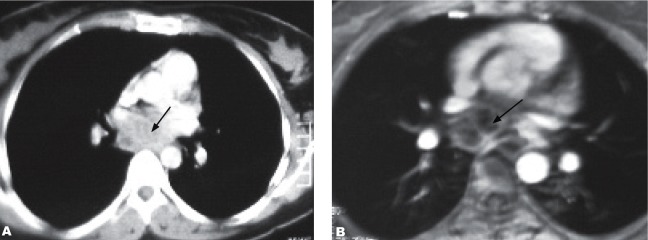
A 30-year-old female patient with mediastinal tuberculous lymphadenitis presenting as a mediastinal mass with dysphagia. A. Thoracic CT shows a 5.5×4.8×3.1 cm mass with subcarinal localization in the middle mediastinum with a multilocular cystic/necrotic appearance and multiple septa. B. MRI shows a subcarinally located 5.5×4,8×3.1 cm mass in the middle mediastinum with a multilocular cystic/necrotic appearance and multiple septa.
